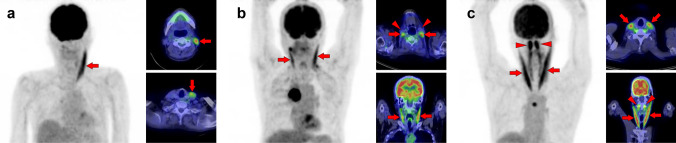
Sternocleidomastoid muscle.* The sternocleidomastoid is one of the most frequently visualized muscles on [^18^F]FDG PET. However, unilateral isolated ones may be occasionally hard to interpret for beginners (a, arrow). There are two branches in the sternocleidomastoid muscles, which originate from the sternum and the clavicle. The sternal branch is more commonly visualized but is rare for the clavicular branch (b, arrows) without uptake in the sternal branch (b, arrowheads). The longus capitis and longus colli muscles (c, arrowheads) are often observed together with the sternocleidomastoid muscle (c, arrows)
